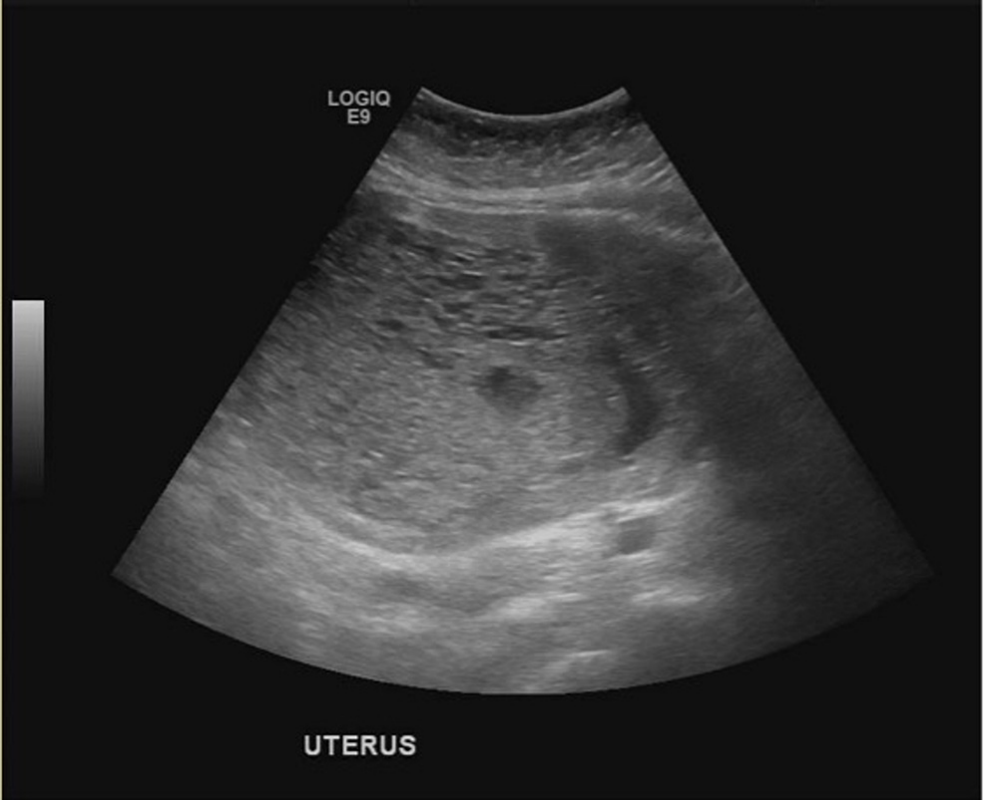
Transabdominal ultrasound showing an enlarged uterus with cystic spaces and absent fetal parts.
